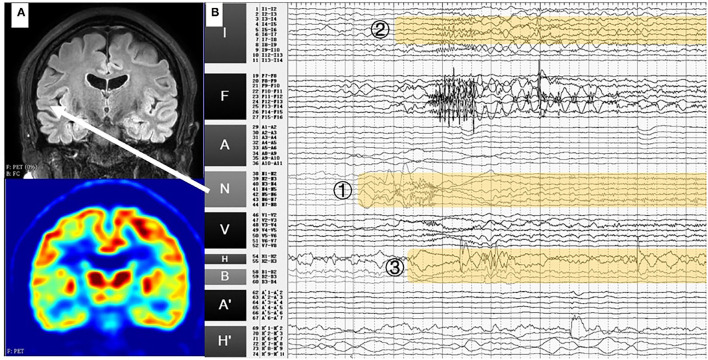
(A) T1-weighted MRI and PET results of case 1. No abnormality was found in MRI, while hypometabolism was identified in the right superior temporal gyrus. (B) Stereoelectroencephalography (SEEG) signals in the ictal period. Fast activities originated from the right superior temporal gyrus (N1-8) and spread rapidly to the right insular lobe (I3-8) and hippocampus (H1-3, B1-3). White arrow: the location of the electrodes N in MRI (right superior temporal gyrus).
